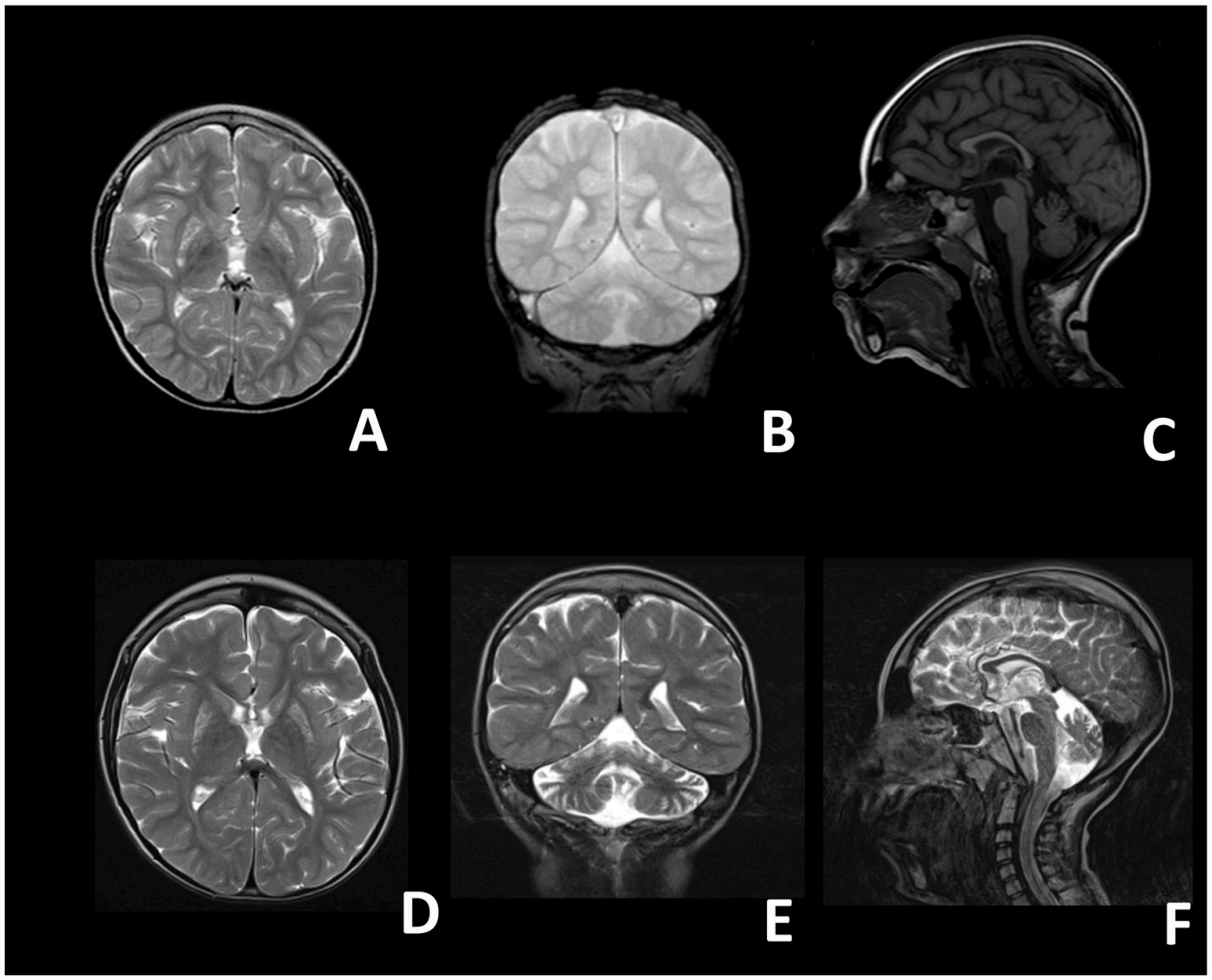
Brain magnetic resonance images of the proband. (A–C) Images taken at 4 years; (D–F) images taken at 8 years. (A) T2 axial, (B) T2 coronal, (C) T1 sagittal, (D) T2 axial, (E) T2 coronal, (F) T2 sagittal. A-D: bilateral inhomogeneous lesions of the Putamen and of the Caudatum. (B–E,F) Progressive cerebellar (vermian and hemispheric) atrophy. (C–F) Thin corpus callosum.
